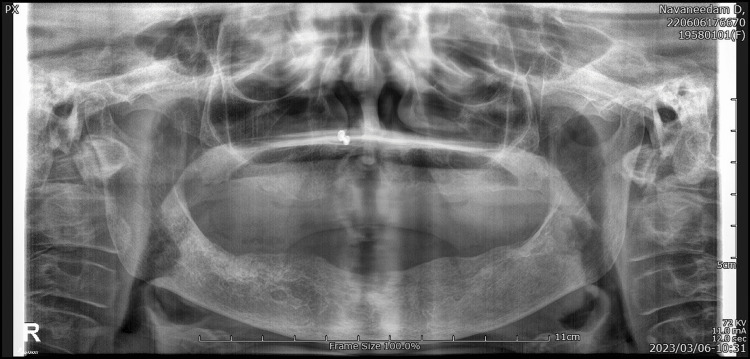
Post-operative radiograph Represents the post-operative orthopantomogram taken after eight months shows the completely edentulous upper and lower arch.
